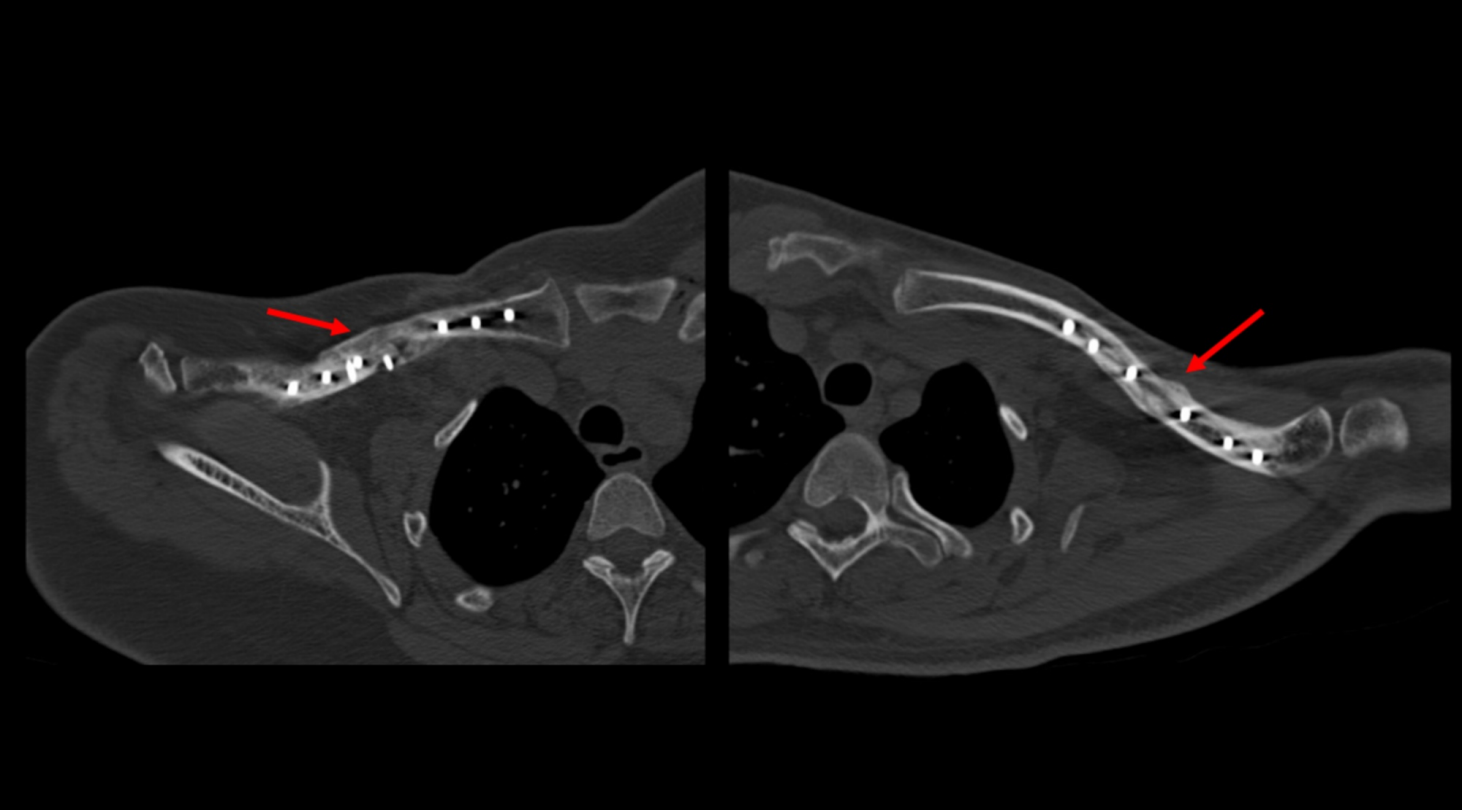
CT scans and bone union achieved after surgery CT: computed tomography Red arrow: signs of bone union
